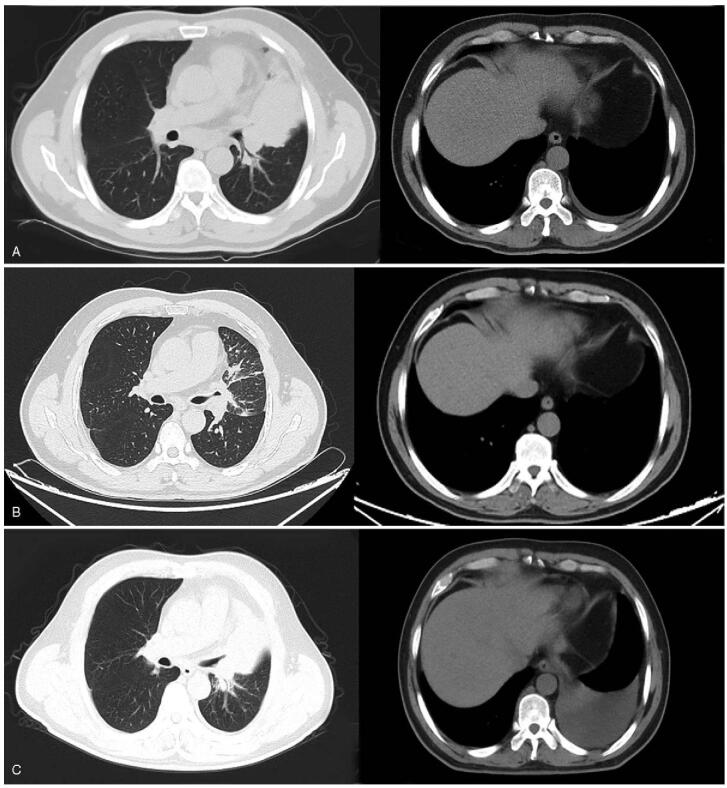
Chest CT imaging results for the case 2 during treatment with dacomitinib. (A) Chest CT showed a high density mass in the left superior lobe accompanied by enlarged mediastinum, left hilum lymph nodes; a mild left pleural effusion was also detected at diagnose (April 20, 2020). (B) Three months after treatment, chest CT showed a remarkable reduction in the lung lesions, and pleural effusion was completely disappeared, the efficacy was evaluated as a PR (July 25, 2020). (C) A repeat CT scan indicated progression of lung lesions, the left pleural effusion occurred again, and the mass in the left upper lobe of the lung was enlarged (December 30, 2020). CT = computed tomography, PR = partial remission.
